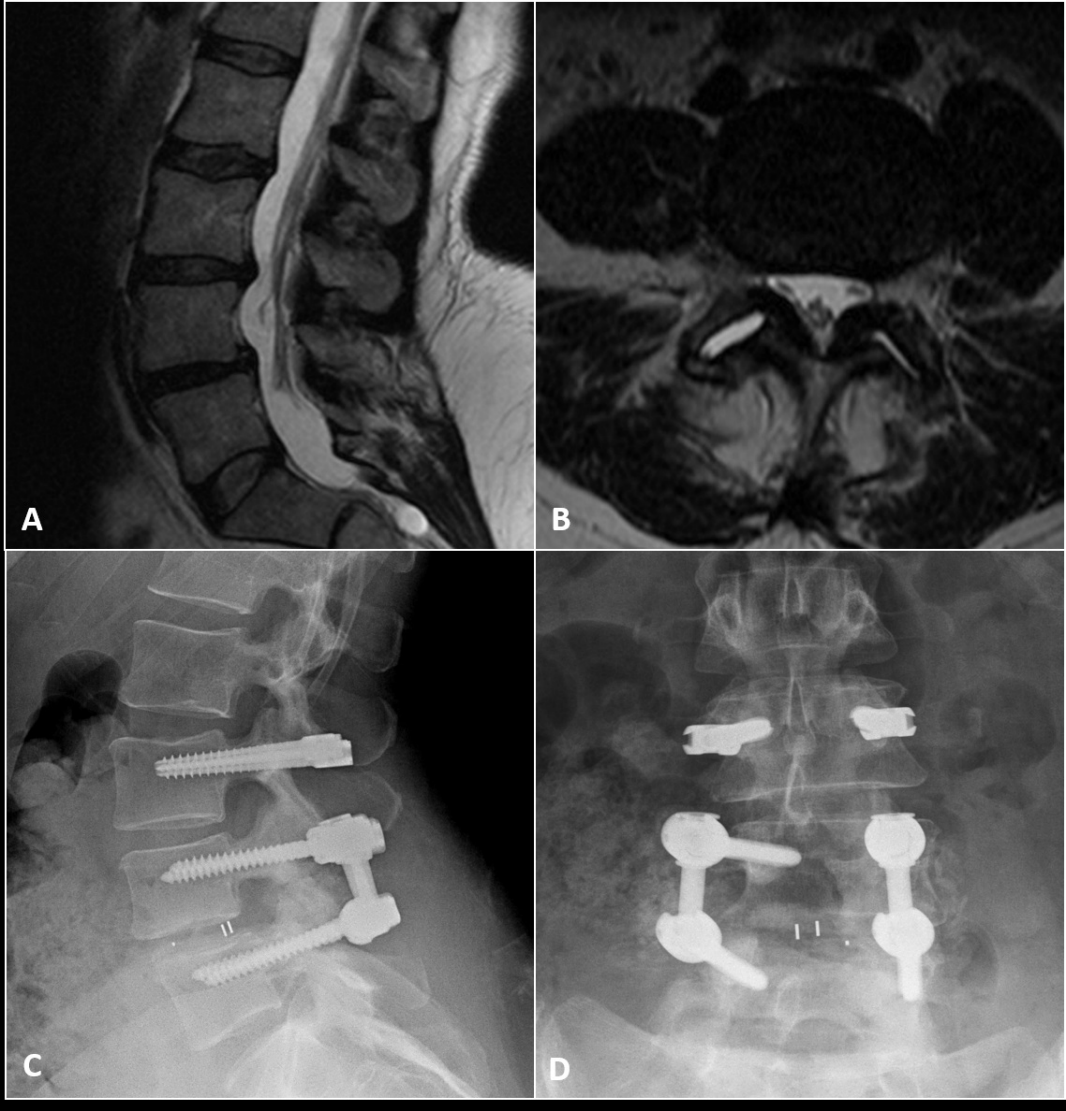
Case example of a 54-year-old woman with disabling low back and leg pain in whom nonsurgical therapy had failed. A: Preoperative sagittal T2-weighted MR image reveals a Grade I spondylolisthesis of L4-5 with a large L3-4 disc herniation. B: Preoperative axial T2-weighted MR image through the L4-5 disc space reveals bilateral facet disruption. It was elected to perform an L4-5 arthrodesis and L3-4 dynamic stabilization procedure. C: A postoperative lateral radiograph demonstrating the hybrid construct with a dynamically stabilized segment (L3-4) above a rigid fused segment (L5-S1). An interbody spacer was placed at L4-5. In all cases, a lordotic rod was implanted in order to preserve and/or restore normal lumbar lordosis. Every attempt was made when implanting the bumper to lock the patient into a lordotic posture. D: Postoperative AP radiograph demonstrating the DTO hybrid construct.
